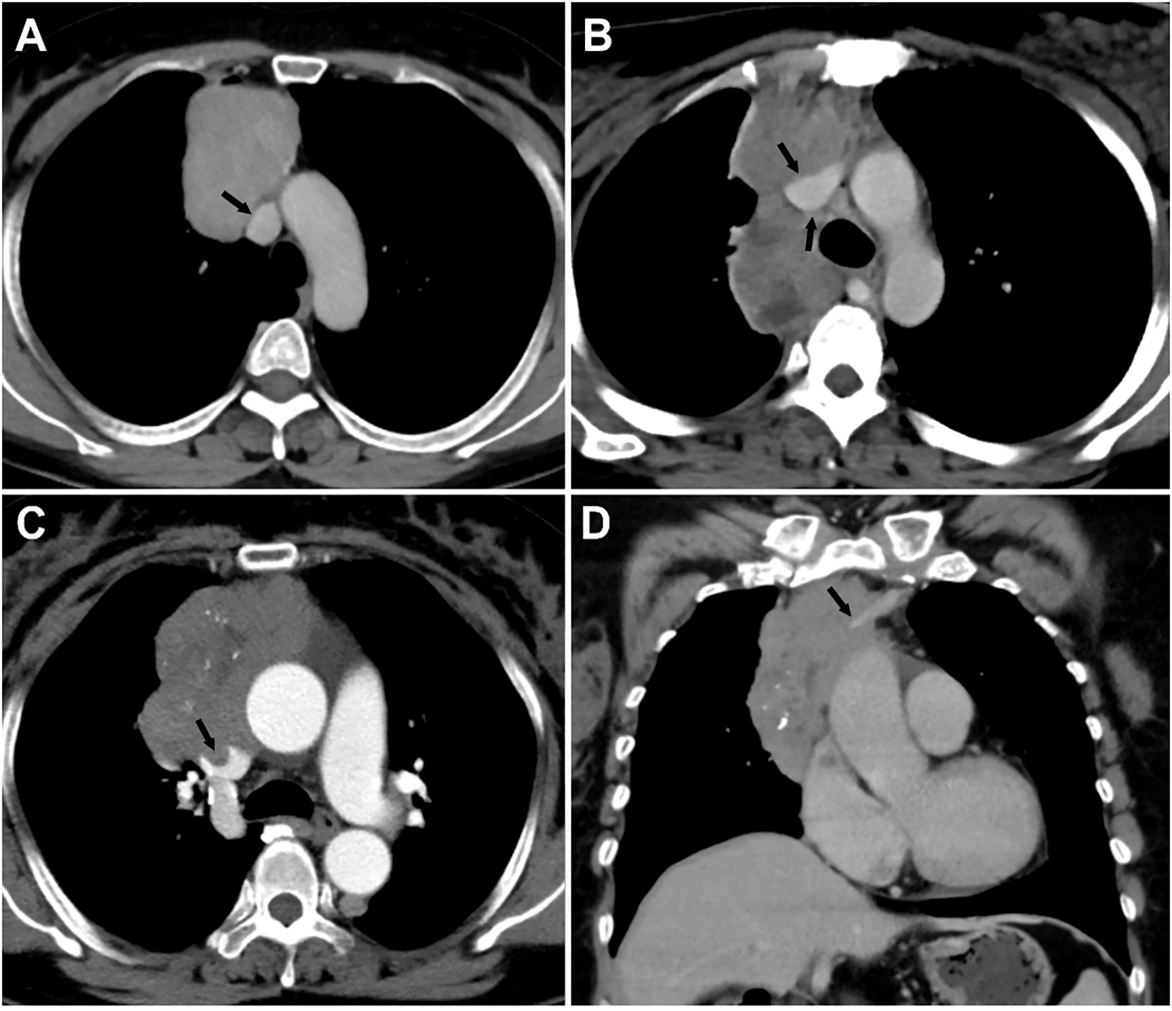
Grading of major mediastinal vein invasion on contrast-enhanced CT images. (A) Grade I, <50%. A 49-year-old woman with type AB thymoma. Tumor abutment degree with adjacent superior vena cava (SVC) is less than 50% (arrow) on axial venous phase enhanced CT image. (B) Grade II, ≥50%. A 30-year-old woman with type B3 thymoma. Tumor abutment degree with adjacent SVC is more than 50% (arrow) on axial venous phase enhanced CT image. (C, D) Grade II, ≥50%, with a filling defect in the SVC and occlusion of the left innominate vein. A 59-year-old woman with type B2 thymoma. Tumor abutment degree with adjacent SVC is more than 50% with filling defect in the SVC (arrow) on axial enhanced CT image (C). Coronal venous phase enhanced CT image (D) reveals the occlusion of the left innominate vein (arrow).
